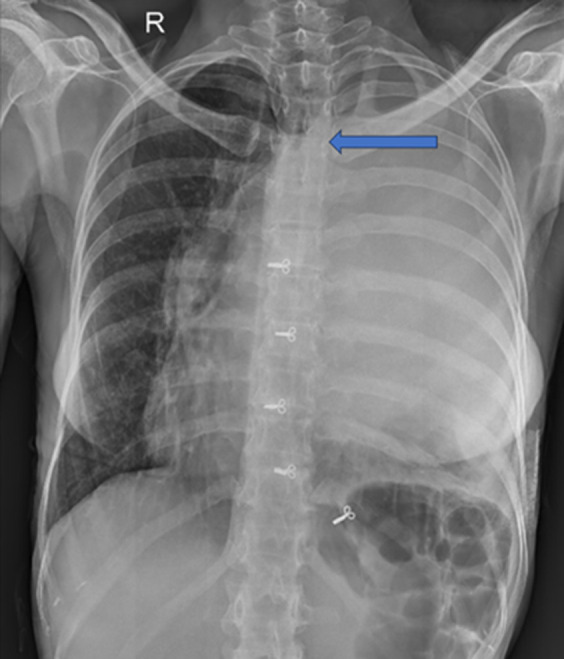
chest X-ray showing left opaque hemithorax with trachea pushed to the right side and a blue arrow showing left bronchus cut-off sign
